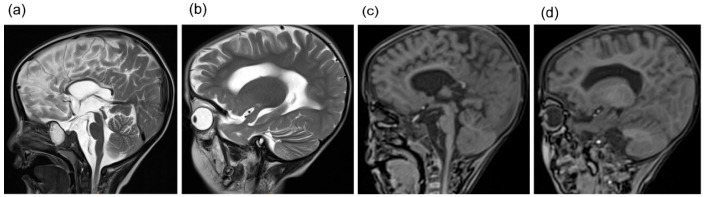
MRI of the brain. (a–d) Significant thinning of the corpus callosum and structural changes in the anterior horns of the lateral ventricles bilaterally.
